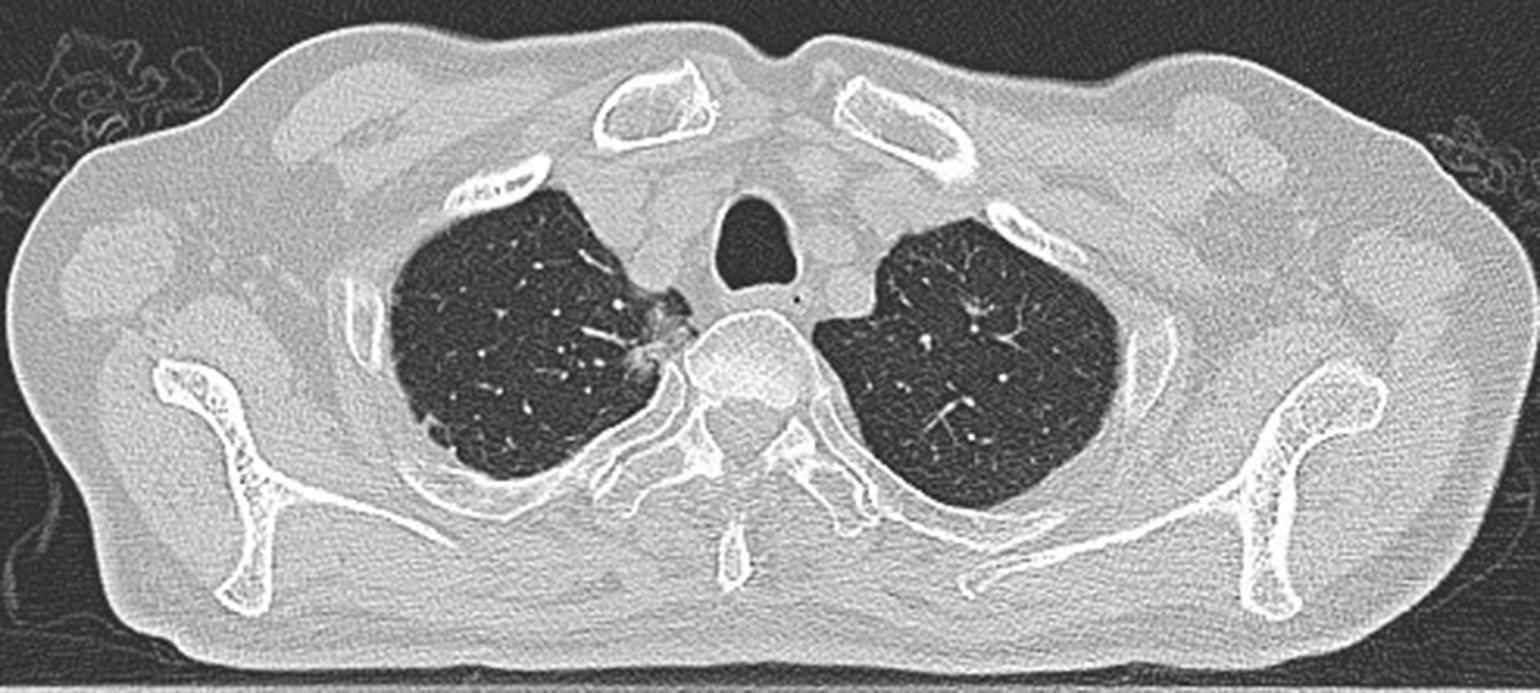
Preoperative scan: Preoperative CT showed a nodule in the right upper lobe. Invasive adenocarcinoma of the right upper lobe of a 78-year-old man.
